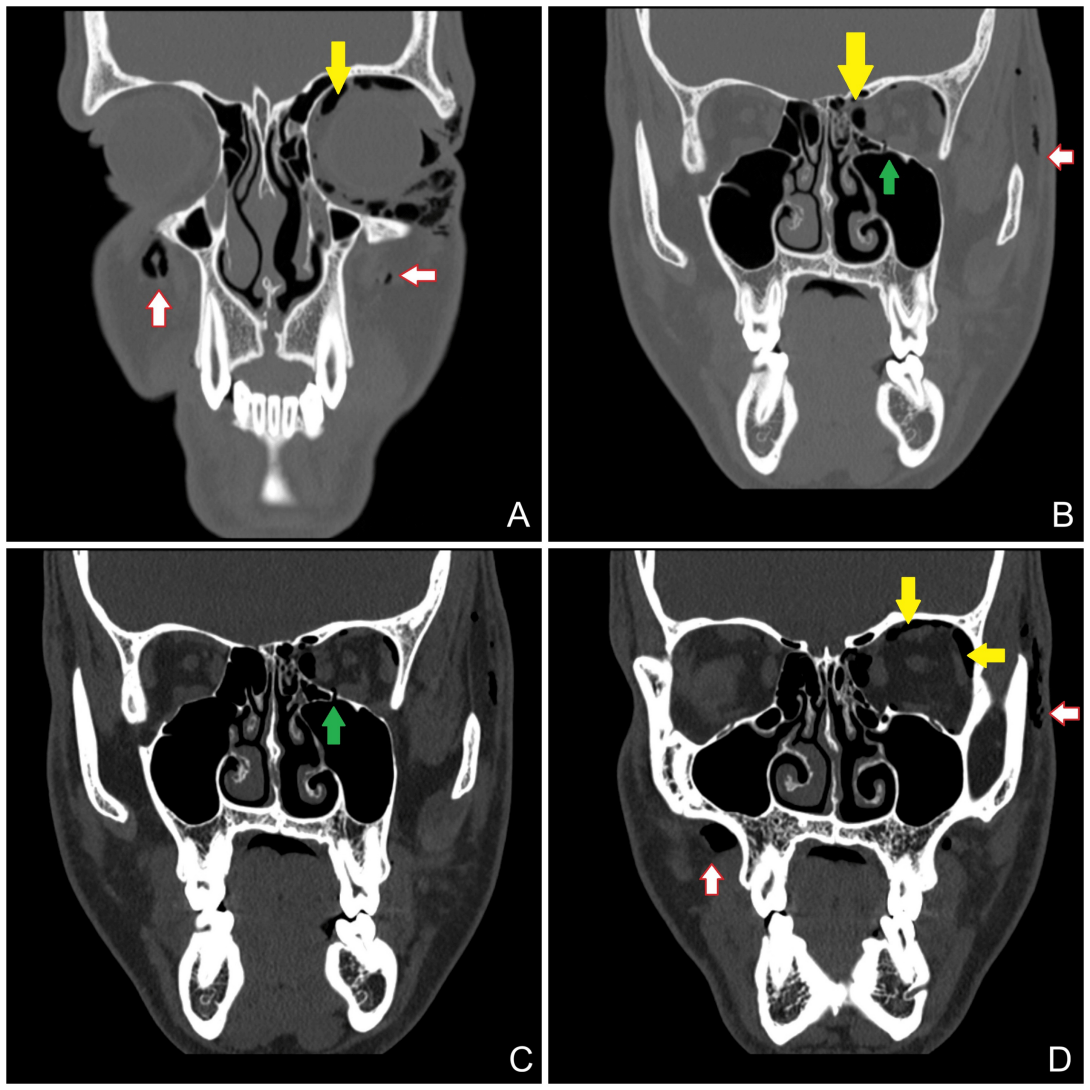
Coronal view computed tomography (CT) scan of the face demonstrating orbital (yellow arrows), subconjunctival, and subcutaneous emphysema (white arrows) associated with a small fracture (green arrows) of the left medial orbital wall
